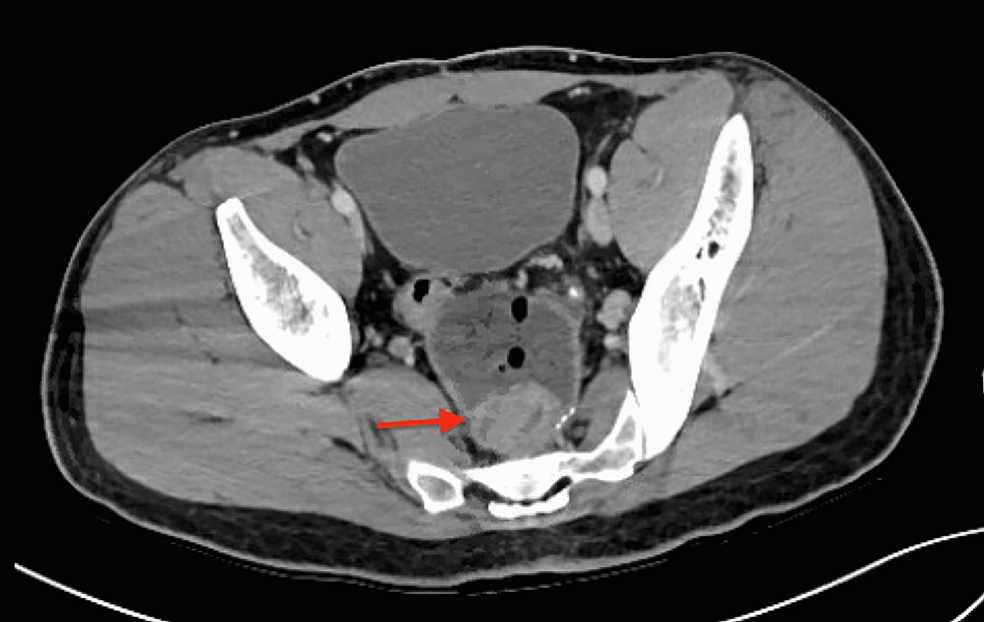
CT of the abdomen/pelvis with contrast demonstrating 2.7-cm rectal mass (red arrow). CT: computed tomography
